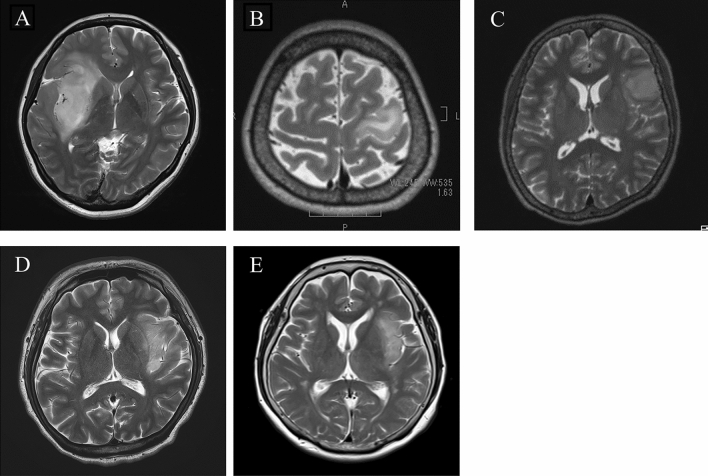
Preoperative axial T2-weighted MRI, showing a high-intensity area with a well-defined tumor border of the right insular tumor (Case 1) (A), of the precentral gyrus (Case 2) (B), of the left inferior frontal gyrus tumor (Case 3) (C), of the left insular tumor (Case 4) (D) and, of the left insular tumor (Case 5) (E).
